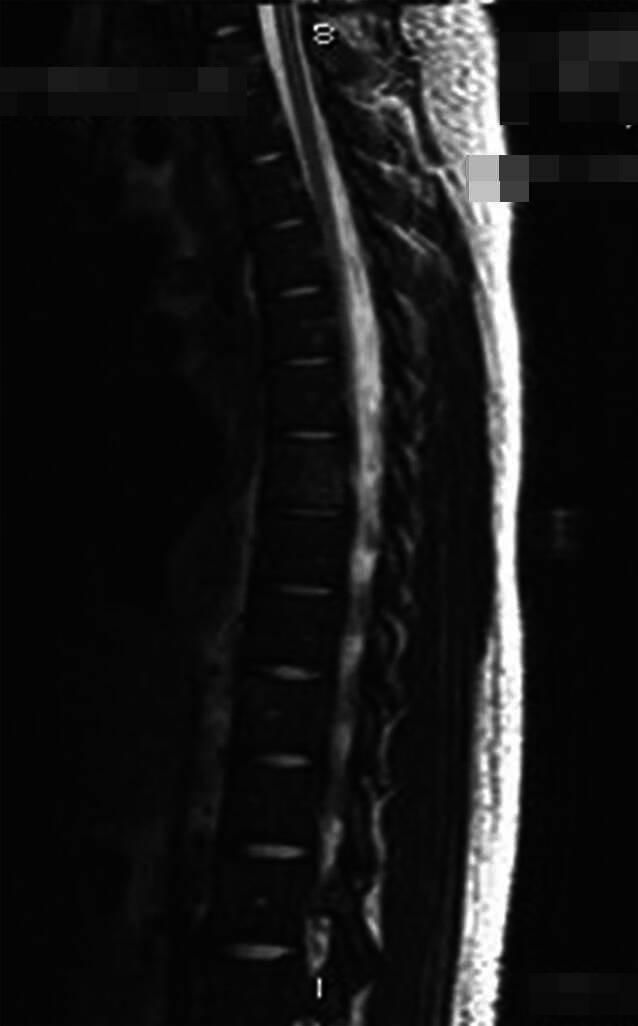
MRI revealing multiple infiltrative lesions involving thoracic and lumbar vertebral bodies
